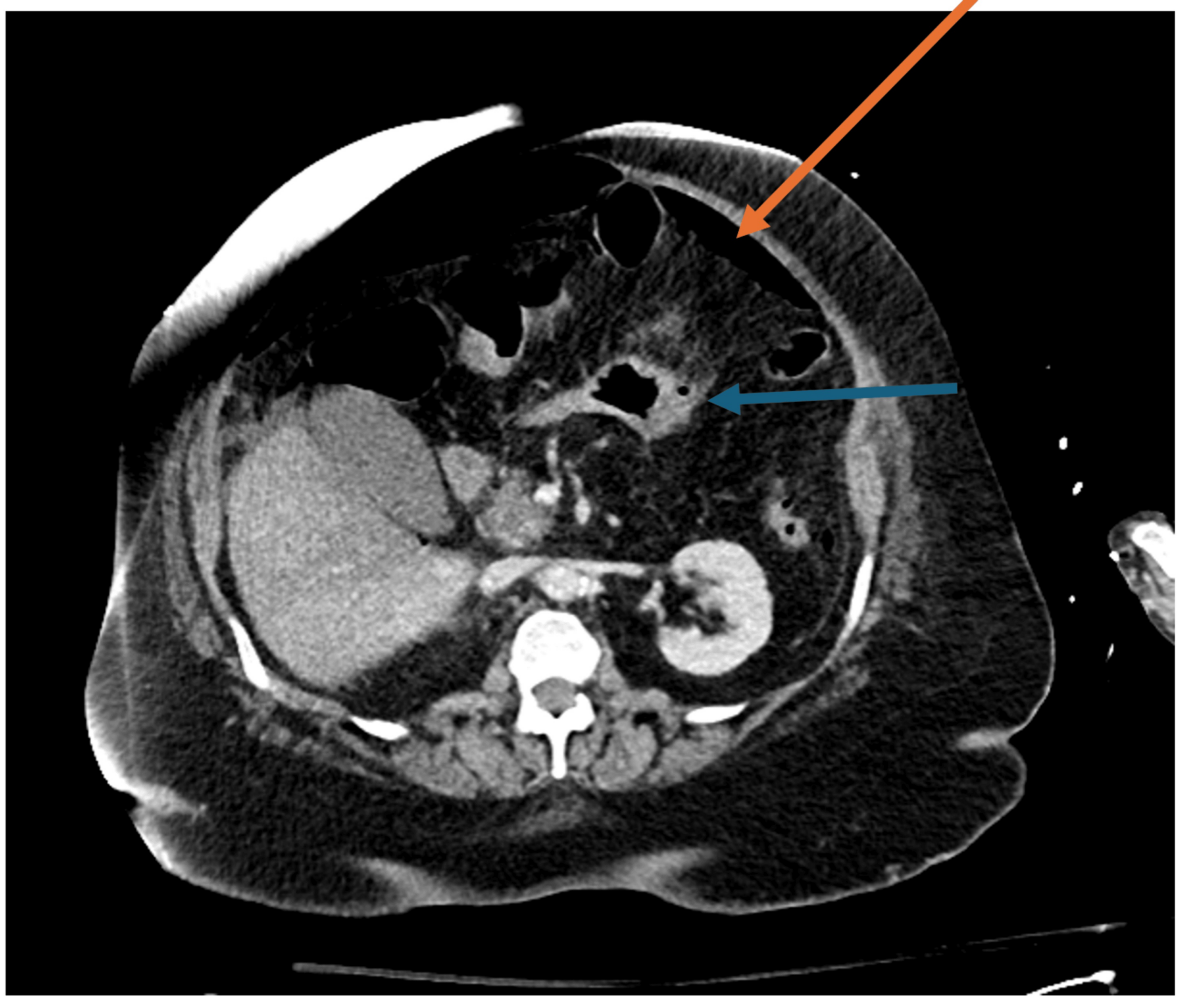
Computed tomography demonstrating gastric thickening with massive pneumoperitoneum outside of gastric wall. Orange arrows point to the pneumoperitoneum. Blue arrows point to gastric thickening.
